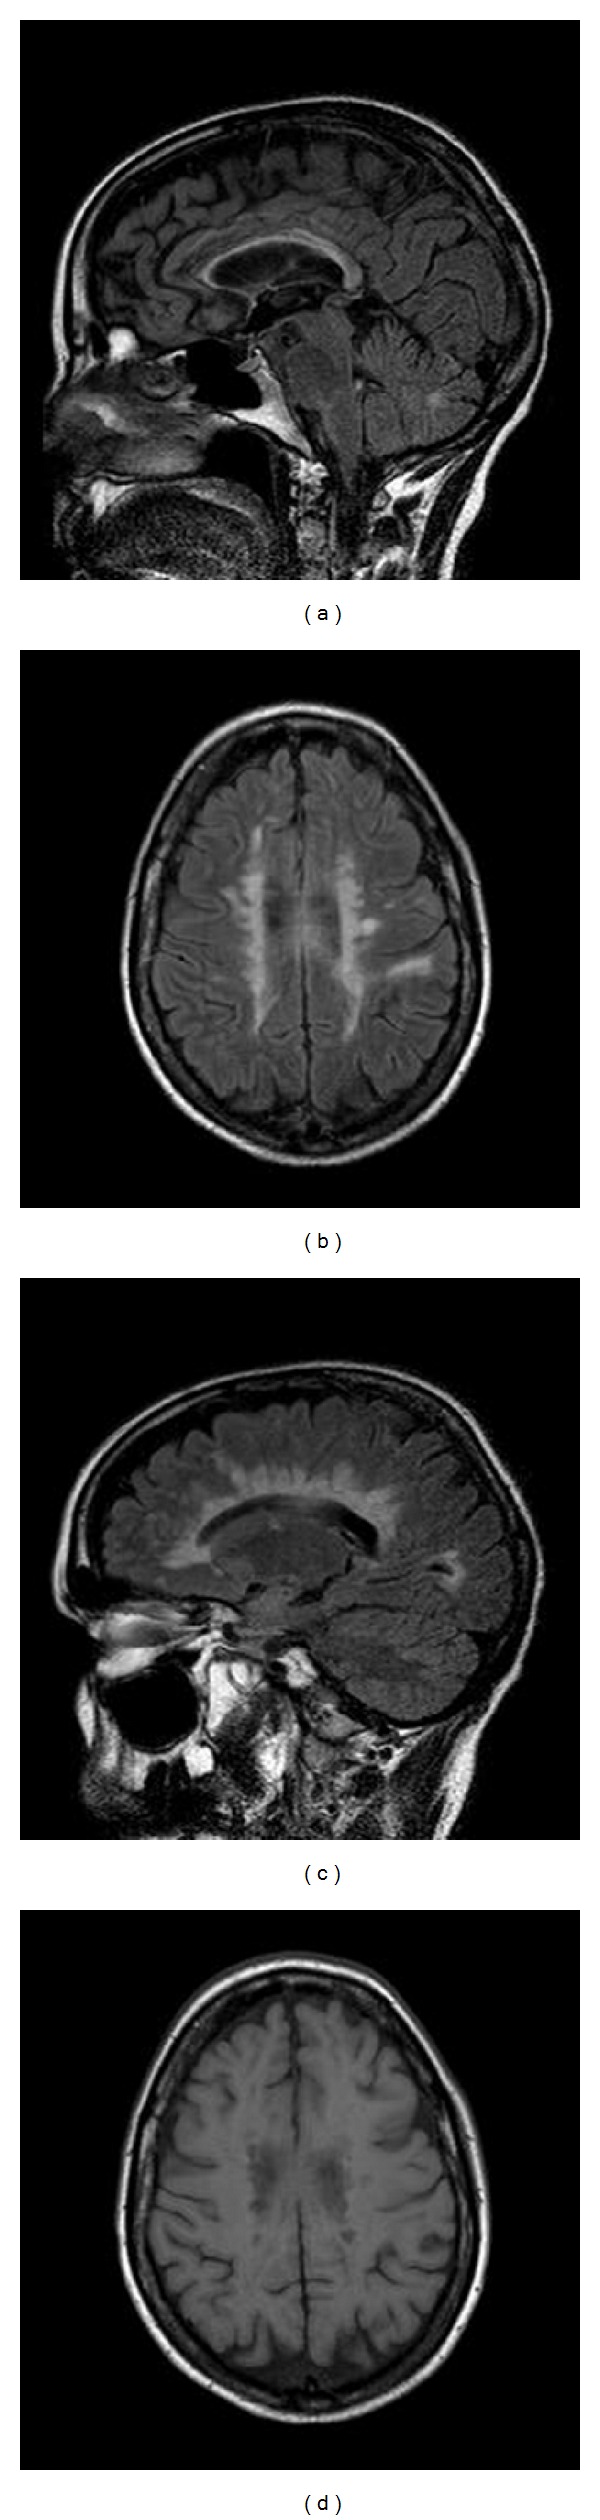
Sagittal (a), axial (b), and parasagittal (c) FLAIR MRI scan showing corpus callosum involvement. T1 axial scan (d) showing the presence of numerous T1 hypointense lesions (“black holes”).
